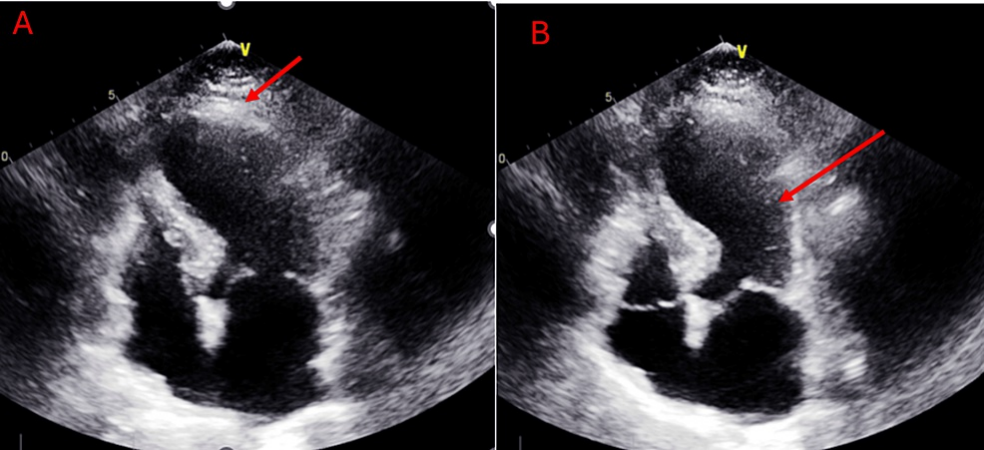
Apical four-chamber view of the heart. (A) Arrow pointing at the apical side of the heart shows signs of apical ballooning and akinesia and (B) arrow pointing at the left chamber of the heart shows ballooning of the left ventricle and a "Jar shape."
